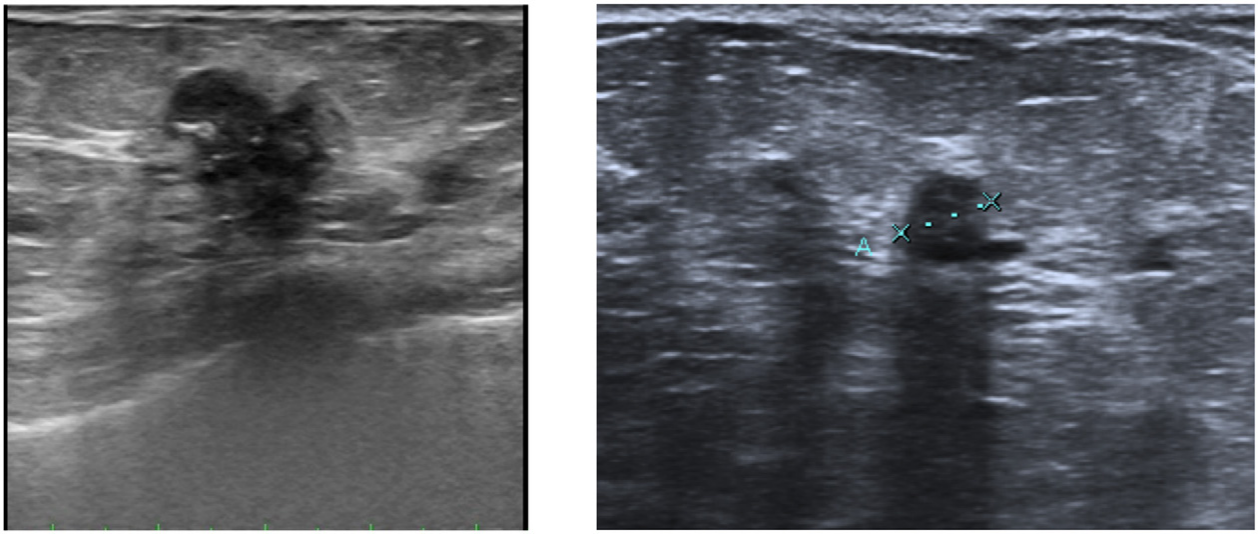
Breast cancer ultrasound images.
